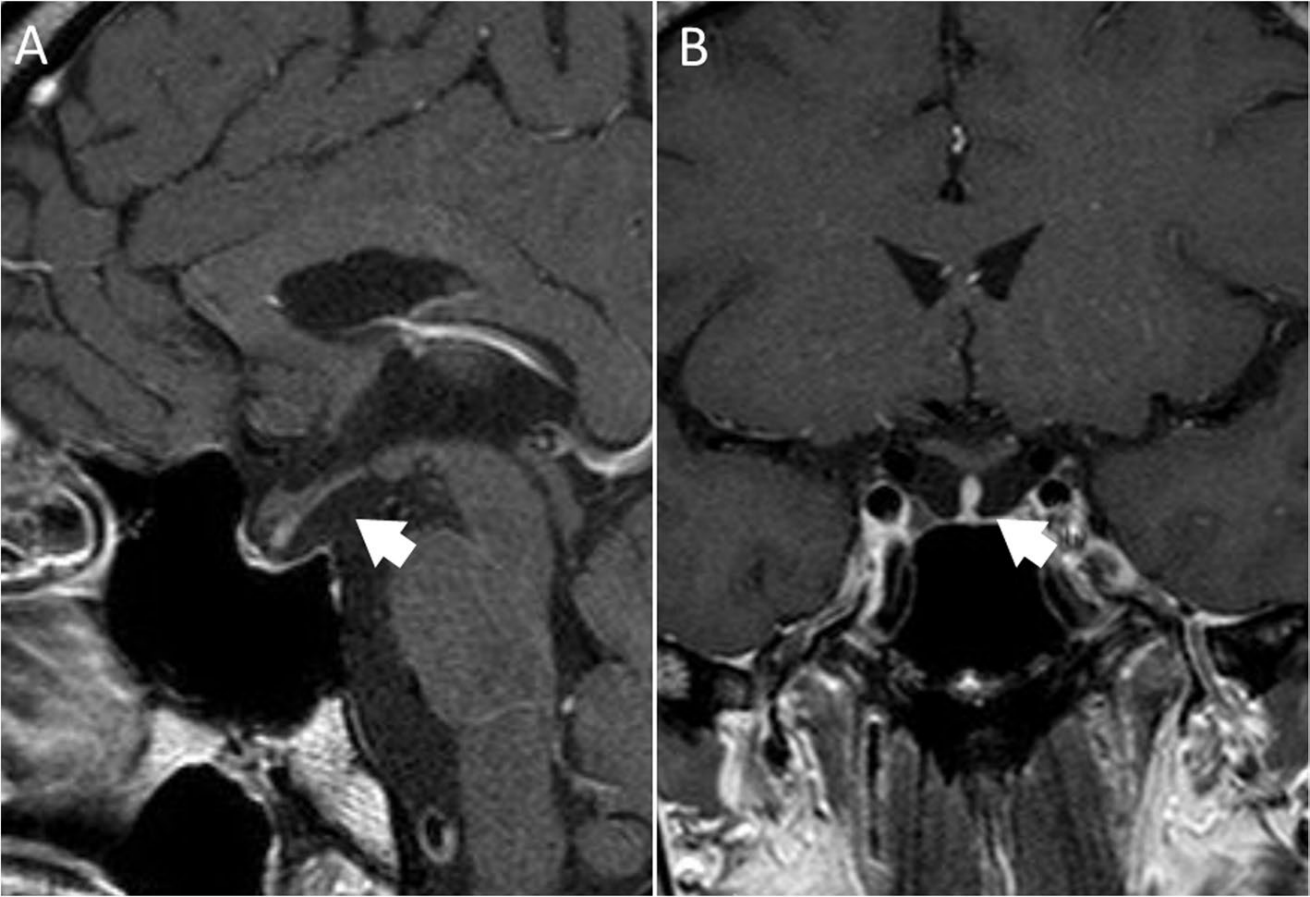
A MR T1-weighted sagittal image reveals cerebrospinal fluid filled into the pituitary fossa (*white arrow*). B MR T1-weighted coronal image showed a thin and flat pituitary gland (*white arrow*)
